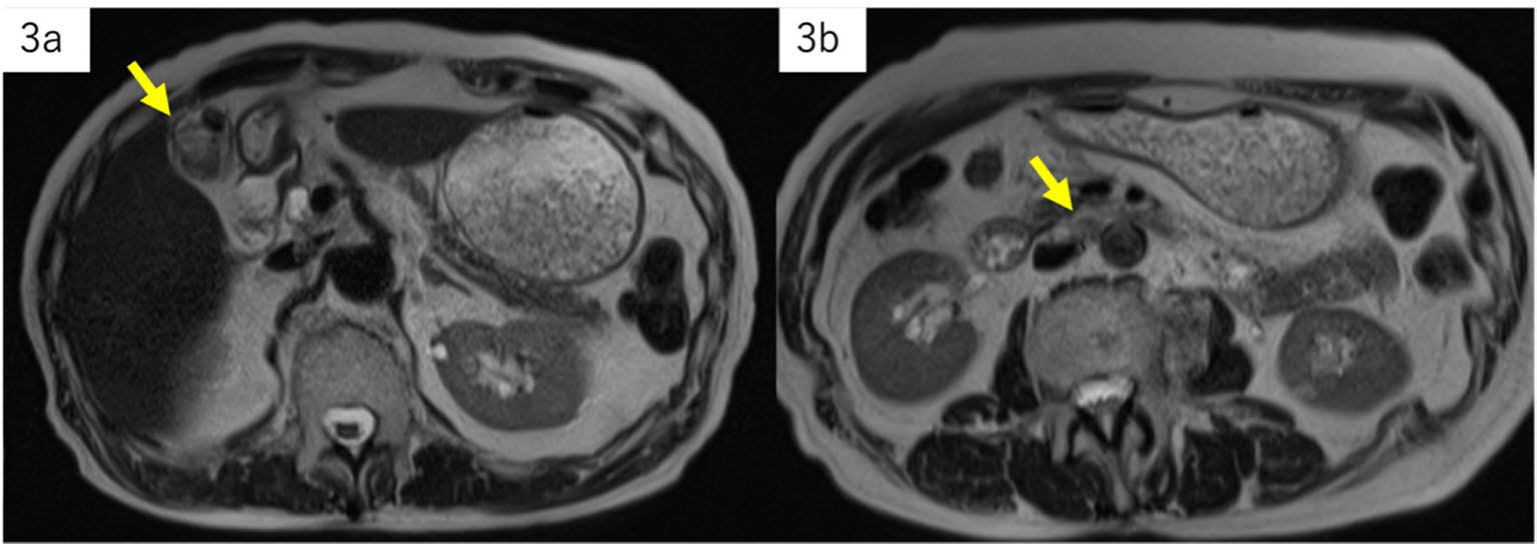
Magnetic resonance imaging. The gallbladder lesion (a) and para‐aortic mass (b) show inhomogeneous signals on T2WI. T2WI, T2 weighted image.
